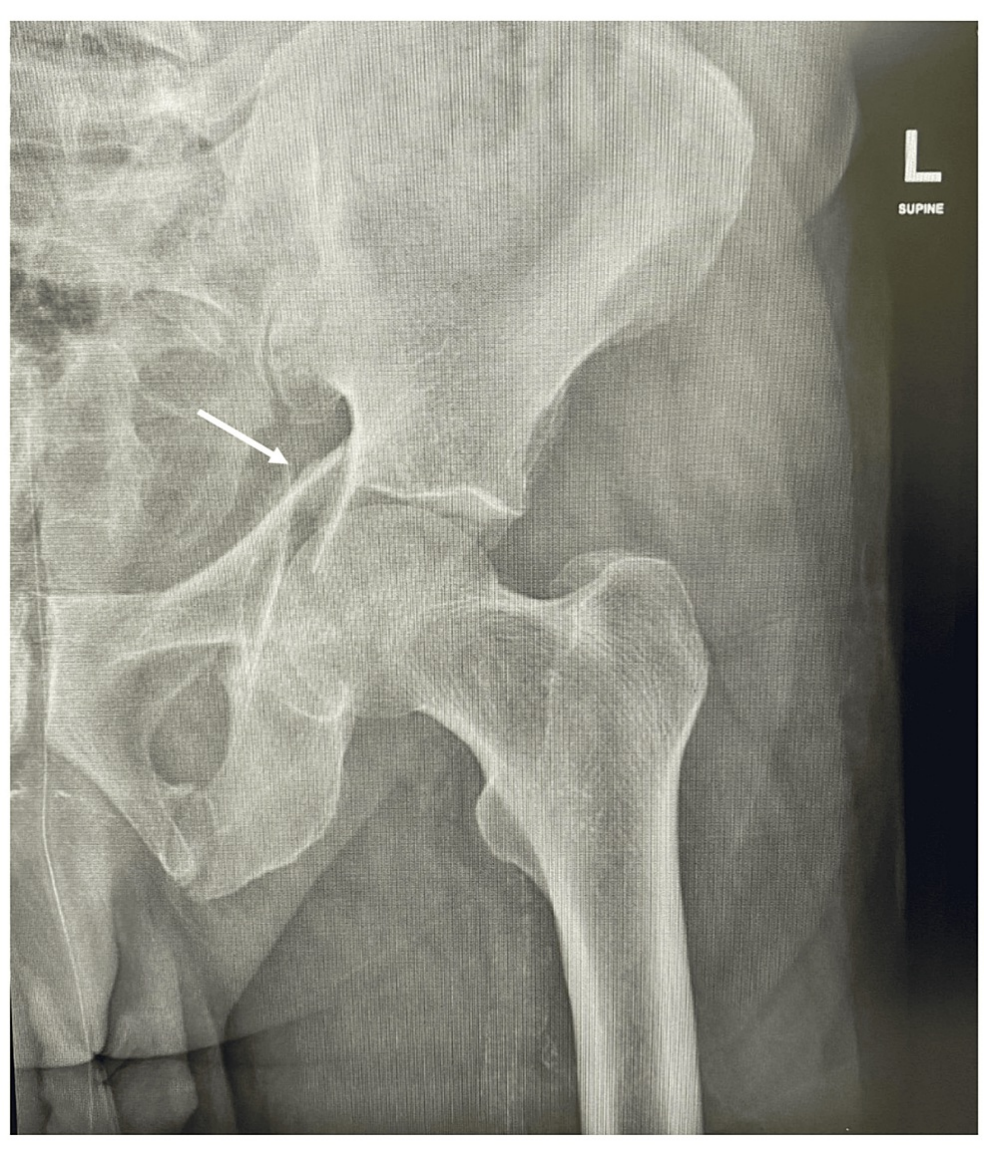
Anteroposterior (AP) x-ray of the left hip showing left acetabulum and inferior pubic ramus fracture.
